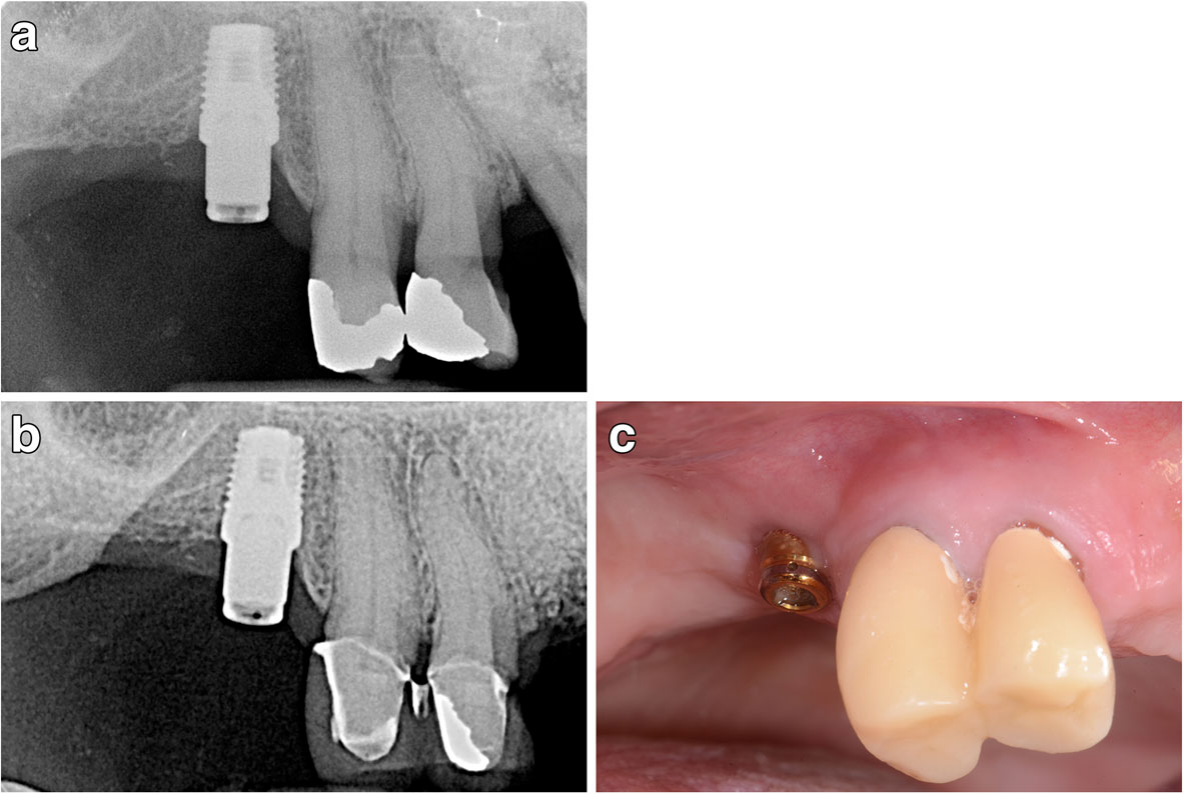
a Initial radiograph exposed at abutment installation on a 5-mm-wide by 7-mm-length dental implant used to help support a removable partial denture for a 71-year-old Caucasian male. b Radiograph of the area taken at 82 months demonstrates good bone stability. c Clinical image of the area 82 months later. Soft tissue remains healthy. The two teeth anterior are in the process of receiving a two-unit fixed splint
